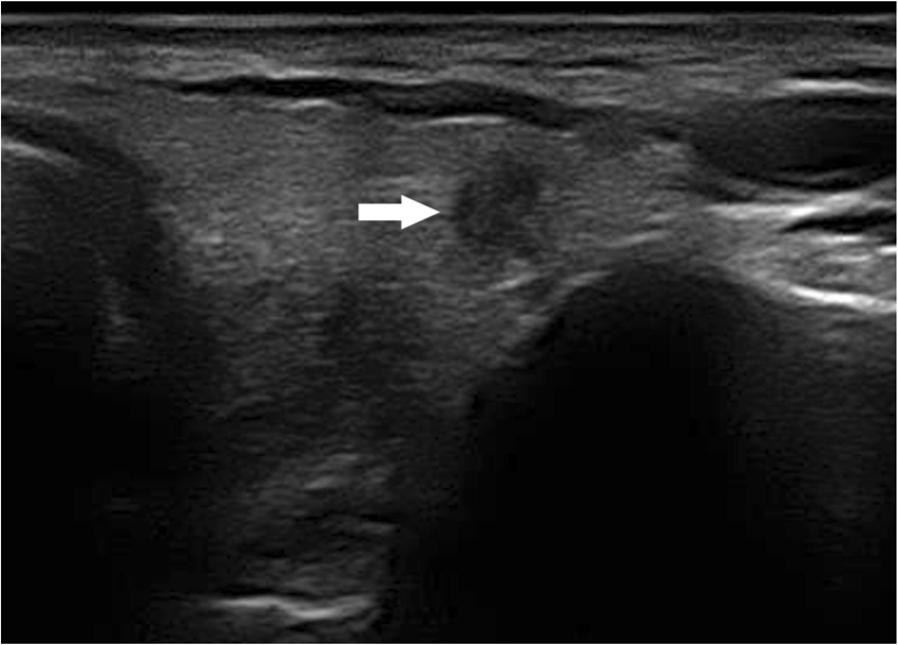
The MTMC nodule transverse sonographic image of a 76-year-old female displayed taller than wide shape, poorly defined margin, and hypoechogenicity
